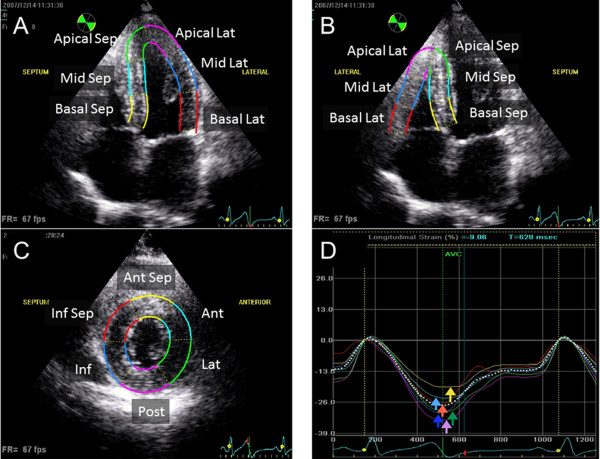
Six-segmental models were created using a tracking algorithm after manual delineation of the endocardial border in the LV apical 4-chamber view (A) and RV apical 4-chamber view (B), and the short axis view for the left ventricular circumferential and radial functional measurements (C). Right bottom panel shows time-strain curves RV apical 4-chamber view for the assessment of longitudinal myocardial systolic function. Solid colored lines indicate corresponding segmental strain curves, and the white dotted line indicates global strain curves (D).
